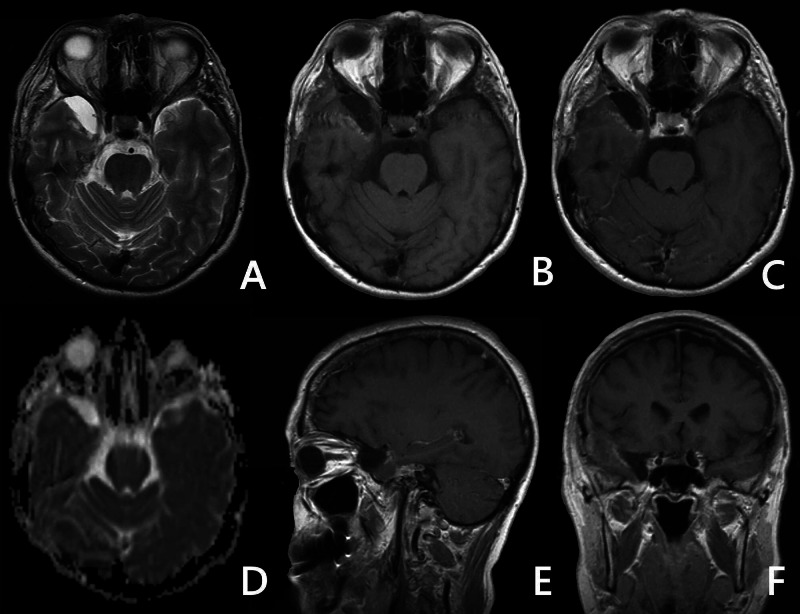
Preoperative MRI study (T2, T1, and T1 + C, DWI) A, B, C: the space-occupying lesion of the anterior sections of the middle cranial fossa in the region of the right temporal pole was determined. The lesion had a hyperintense signal at T2, and a hypointense signal at T1 compared to the brain tissue; no contrast uptake was noted. D: DWI shows no restriction. E, F: sagittal and coronal T1+C shows no contrast enhancement MRI: magnetic resonance imaging; DWI; diffusion-weighted imaging
